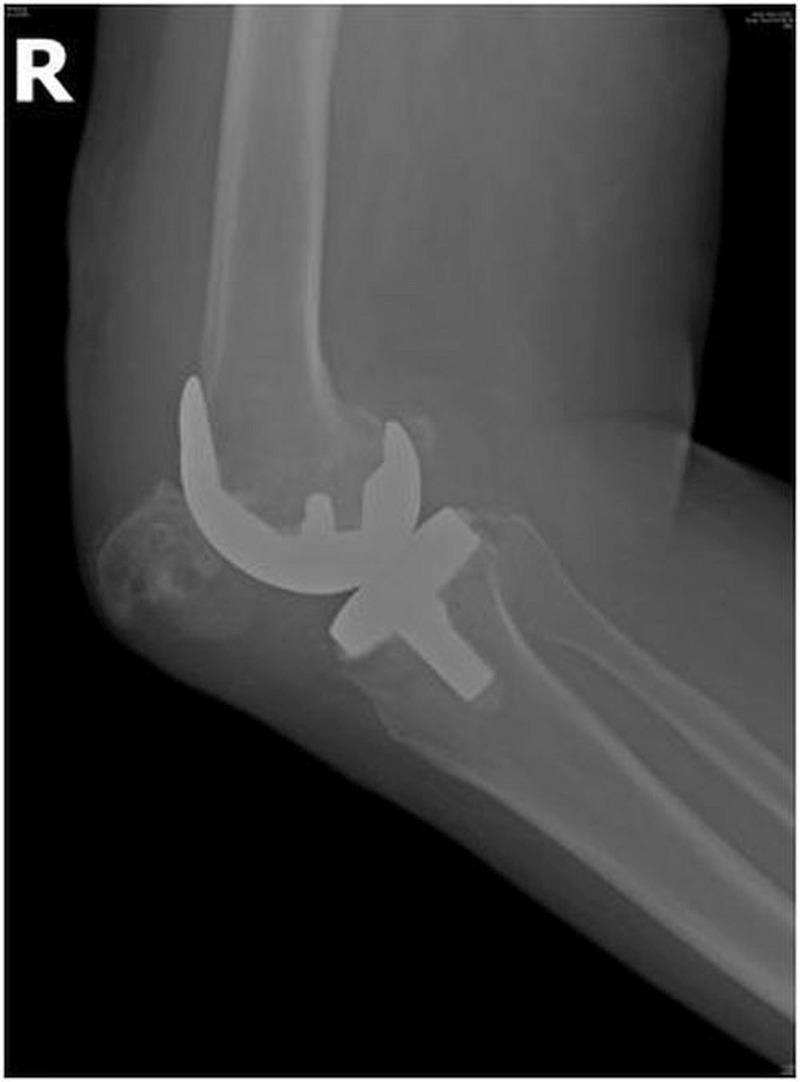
Lateral radiograph of a patient showing lateral patellar dislocation on the right side after TKA.
